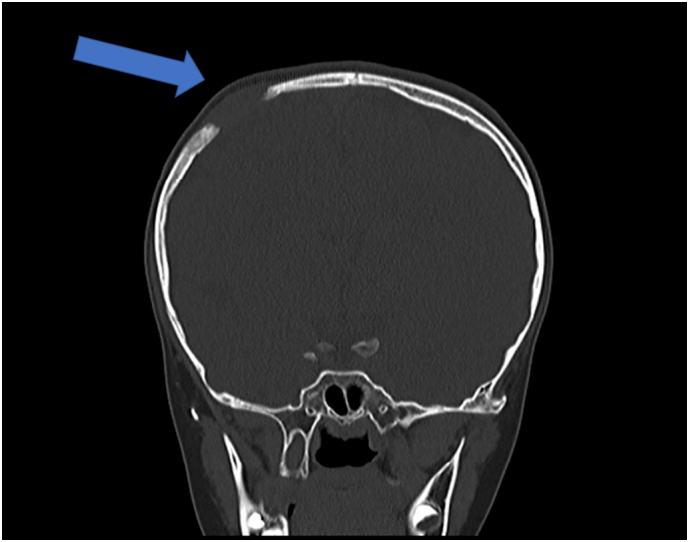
CT scan shows a lytic lesion after treating the infection (Blue Arrow). (For interpretation of the references to colour in this figure legend, the reader is referred to the web version of this article.)
